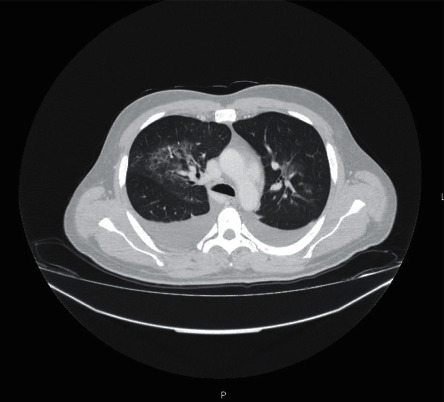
Chest CT with ground glass areas in the left and right pulmonary lobes; bilateral pleural effusion.
